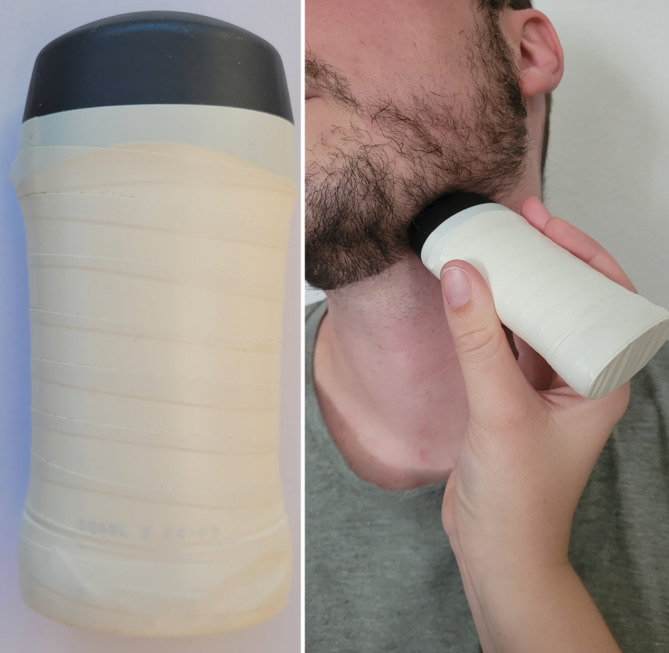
Im Vergleich dazu erlernte die KG die Sweeps an den Tagen 2–4 in 15-minütigen Unterrichtseinheiten am Sonographiegerät. Beide Gruppen führten an Tag 5 die vollständige sonographische Untersuchung am Sonographiegerät im Rahmen einer Lernerfolgskontrolle durch.
